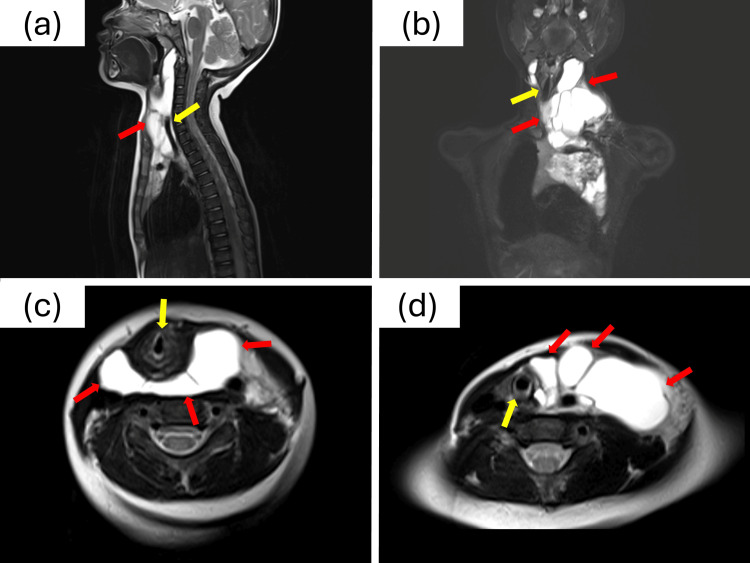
MRI of the multiloculated cervical lymphatic malformation (a) Sagittal T2-weighted image showing the lesion (red arrow) causing significant posterior displacement of the airway from the oropharynx to the trachea (yellow arrow). (b) Coronal T2-weighted image demonstrating the mediastinal extension (red arrows) and rightward deviation of the cervical trachea from the midline (yellow arrow). (c) Axial T2-weighted image at the oropharyngeal level, revealing the lymphatic malformation (red arrows) causing severe airway compression (yellow arrow). (d) Axial T2-weighted image at a lower level, showing circumferential compression of the tracheal lumen (yellow arrow) by the lesion (red arrows).
